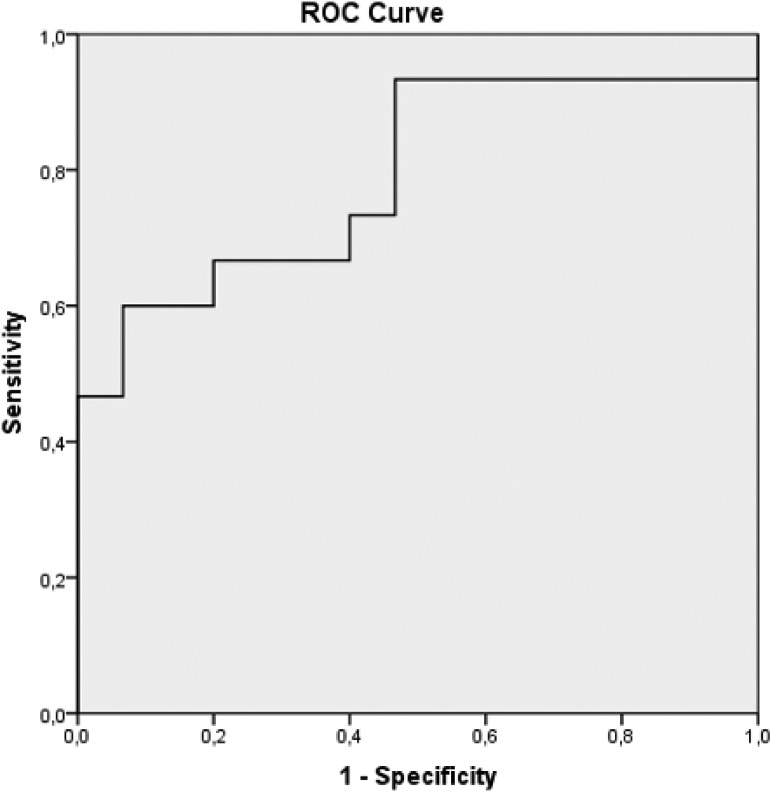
ROC plot with pointwise 95% confidence bounds with the malign and benign pleural effusion CT images train and test with computer-aided decision system. AUC: 0.791; p-value = 0.007.
